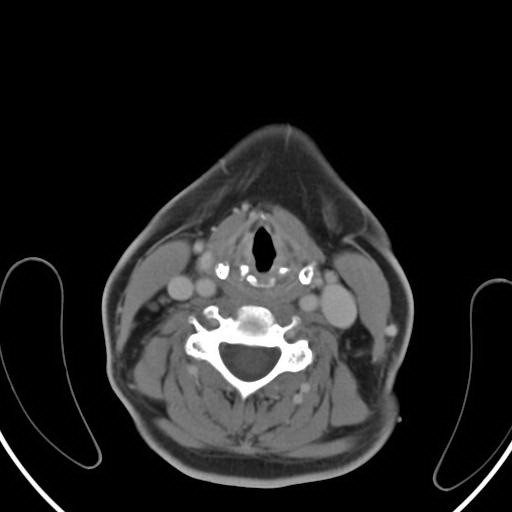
Cervical region CT scan, one month after chemotherapy, negative for residual mass
